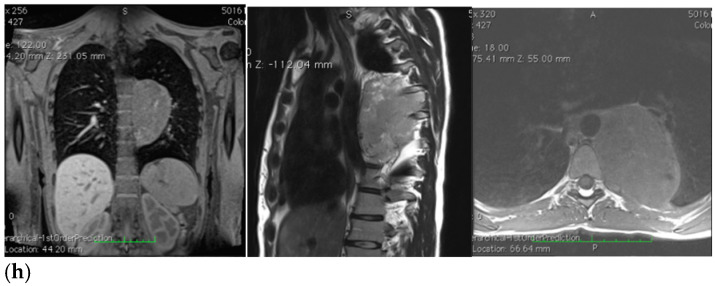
(a–h) Imaging assessments for pre-surgical evaluation. (a) X-ray scans. (NOTE: Voluminous opacity in the posterior mediastinum on the left, with a major axis of about 12 cm). (b) Thorax CT scan with Iodixanol. (NOTE: Solid, oval-shaped tumour in the left posterior mediastinum, measuring 8 × 5.5 cm. Anteriorly, the mass pressed the left main bronchus from behind, while medially it displaced the thoracic aorta—sitting directly on the metamers T5 to T8, and compressing the hemiazygos vein. The mass also went through the intervertebral foramen, between T6 and T7, and partially through the VI and VII intercostal spaces; laterally, it caused dystelectasis of the lung parenchyma. The NB had an irregular density, with innumerable small foci of calcification, and areas of slightly blurred impregnation of the contrast medium). (c) Tracheobronchoscopy. (NOTE: Left lower lobe bronchus with a luminal narrowing, resembling an extrinsic compression. In detail: left lower lobe bronchus, left main bronchus, trachea and carina). (d) Thorax MRI with Gadoteridol. (NOTE: Presence of a mass with maximum dimensions of approximately 7.5 × 5.5 cm axially and 10 cm of craniocaudal extension, limited by a plane passing from upper T6 to lower T9. Extension up to the ipsilateral lung, which caused a moderate compression and a slight compressive effect, also on the left sections of the heart (in particular the atrium). Slight anterior displacement of the ipsilateral pulmonary hilum structures; the neoplasm also came into contact with the aorta. The tumour went through the left T6–T7 intervertebral foramen—taking up space at the foraminal level, not at the level of the spinal canal—and it also extended up the corresponding intercostal space; it was compatible with a lesion originating from the nerve sheath arising from the T6–T7 foraminal region). (e) Total-Body PET/CT. (NOTE: Presence of an increased glucose metabolism lesion in the posterior mediastinal area, showing a necrotic portion and a metabolically more active portion, located in the medial side and infiltrating the posterior arch of the 6th left rib). (f) Thorax CT scan with Lopromide. (NOTE: Minor volumetric increase of the known space-occupying lesion, which maintained similar densitometric characteristics, clear limits, and maximum diameters equal to 8.5 × 6 cm on the transverse plane, with a maximum longitudinal extension of 11 cm. The solid component which went through the intercostal space between T6 and T7 was substantially unchanged. Absence of pleural or pericardial effusion). (g) Total-body scintigraphy with metaiodobenzylguanidine. (NOTE: The examination confirmed that the neoplasm was confined to the mediastinum only. The area of greater contrast medium enhancement corresponded to the left hemithorax, in particular, to the left posterior mediastinal region, from T6 to T9). (h) Thorax MRI with Gadoteridol. (NOTE: Slight increase in size of the known space-occupying lesion in the posterior mediastinum (85 × 60 × 103 mm vs. 80 × 55 × 100 mm). At T6–T7 level, the mass occupied some left foraminal canal space. Next to the known lesion, pleural effusion thin layer (maximum thickness of 11 mm)).
